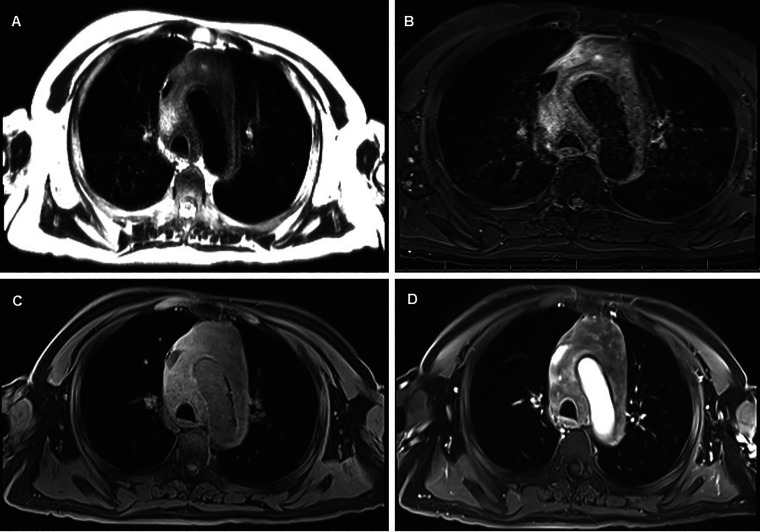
Magnetic resonance imaging (MRI) of the chest demonstrated an infiltrative mediastinal mass with low to intermediate signal intensity on T2 HASTE sequence (A), high signal intensity of T2 STIR sequence (B), intermediate to high signal intensity on T1-weigthed fat-saturated sequence (C), and irregular areas of enhancement on postcontrast sequence (D).
